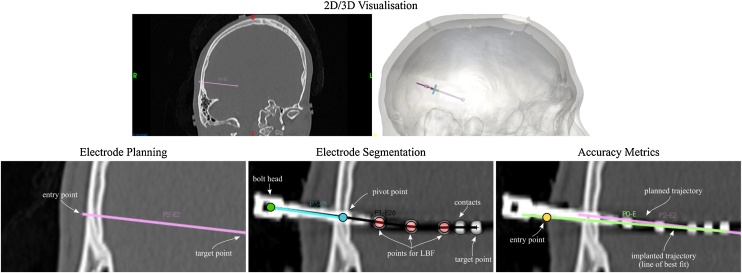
Estimation of SEEG electrode implantation accuracy measures. *Top:* 2D and 3D visualisation of electrode planning showing one electrode. *Bottom:* Close-up view of electrode planning on pre-operative CT image (*left*), segmentation on post-operative CT image (*centre*) and trajectories used for accuracy metrics (*right*). Automated segmentation identifies the position of the bolt head (green circle), pivot point (blue circle) and the position of contacts (black circles). A line of best fit (LBF) is computed for a number of contacts (red circles) and used to compute the position of the implanted entry point (yellow circle). EP and TP localisation errors and angle difference between implanted (green line) and planned (pink line) trajectories are computed. Manual computation of metrics is detailed in Sec. 3.4, whereas our proposed automated approach is detailed in Sec 3.3 as well as Appendix A and B.
